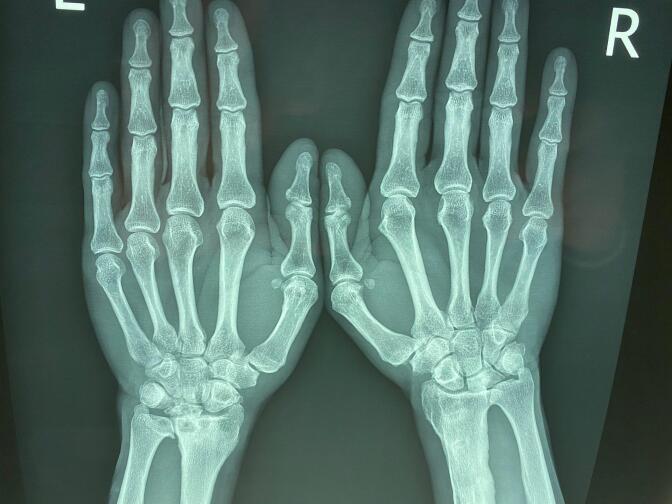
Antroposterior plain radiograph of the right and left wrist in one frame.
